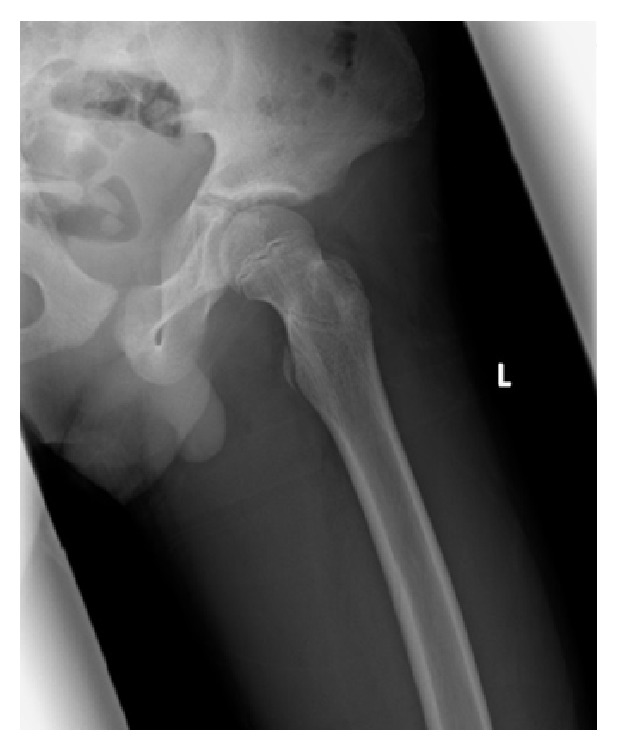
X-ray shows the affected side hip after reduction. The left femoral head is correctly positioned in the acetabulum.
